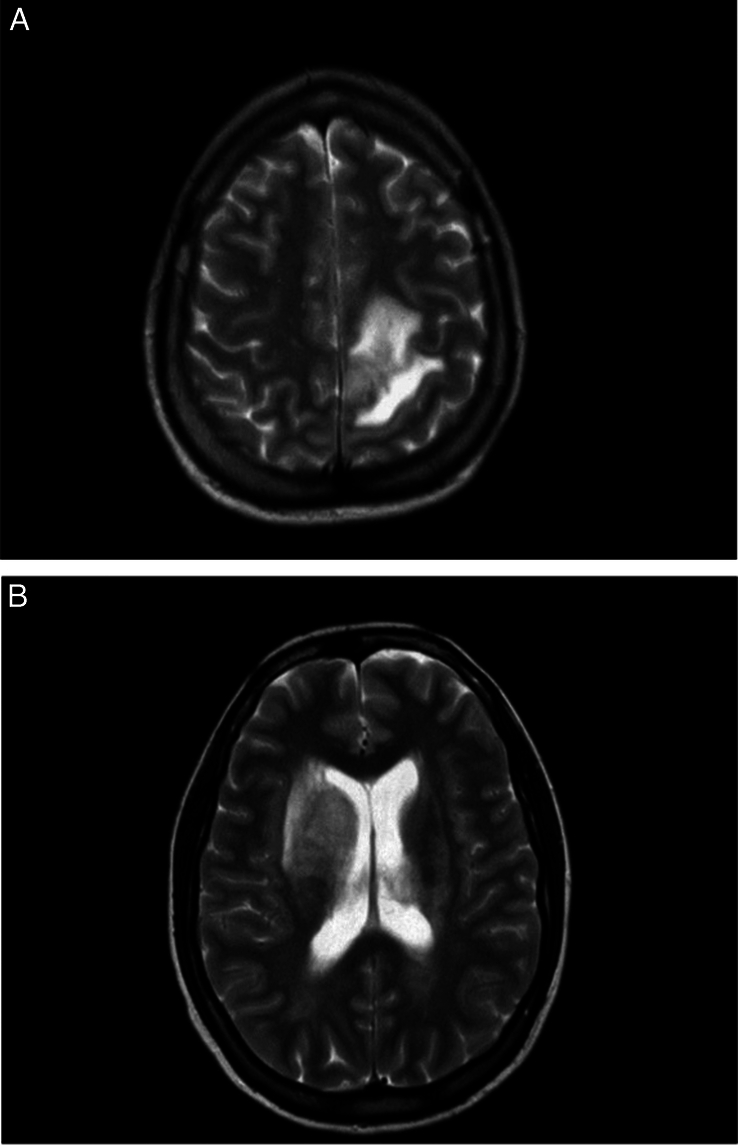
MRI shows multiple bilateral periventricular hyperintensity lesions on T2/FLAIR mainly in the right basal ganglia, both caudate nuclei, left thalamus, and left para-falci grey matter. They were surrounded by white matter oedema. Serpiginous vascular structures were seen after IV contrast gadolinium administration. Fluid-attenuated inversion recovery (FLAIR).
